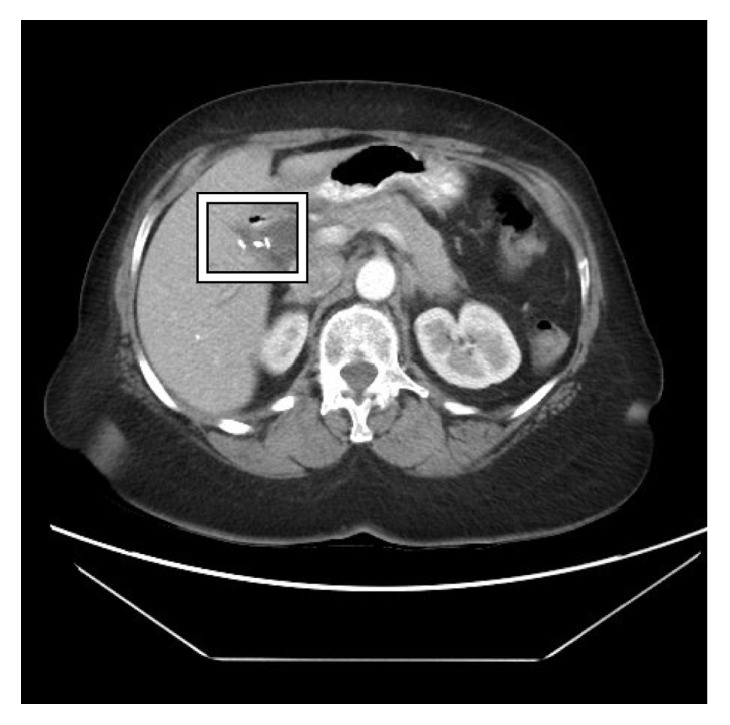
Abdominal CT showing the surgical clips by the cystic duct from the patient's prior cholecystectomy in 2004 (boxed).
